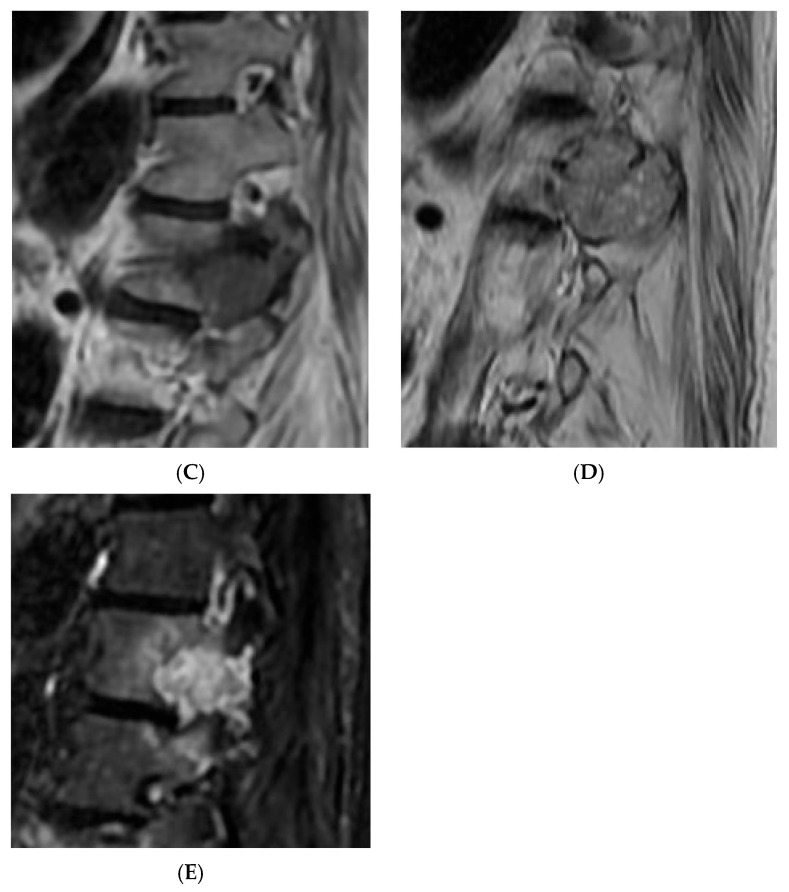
Aggressive spine haemangioma. (A) Axial CT showing lytic lesion within the left-side posterior aspects of the vertebral body with cortical destruction. (B) Axial T2W MRI with the expansile lesion encroaching within the spinal canal and neural foramen. (C) Sagittal T1W MRI. (D) Sagittal T2W MRI and (E) sagittal STIR MRI, demonstrating a more vascular component of the lesion along with expansile component effacing the neural foramen.
